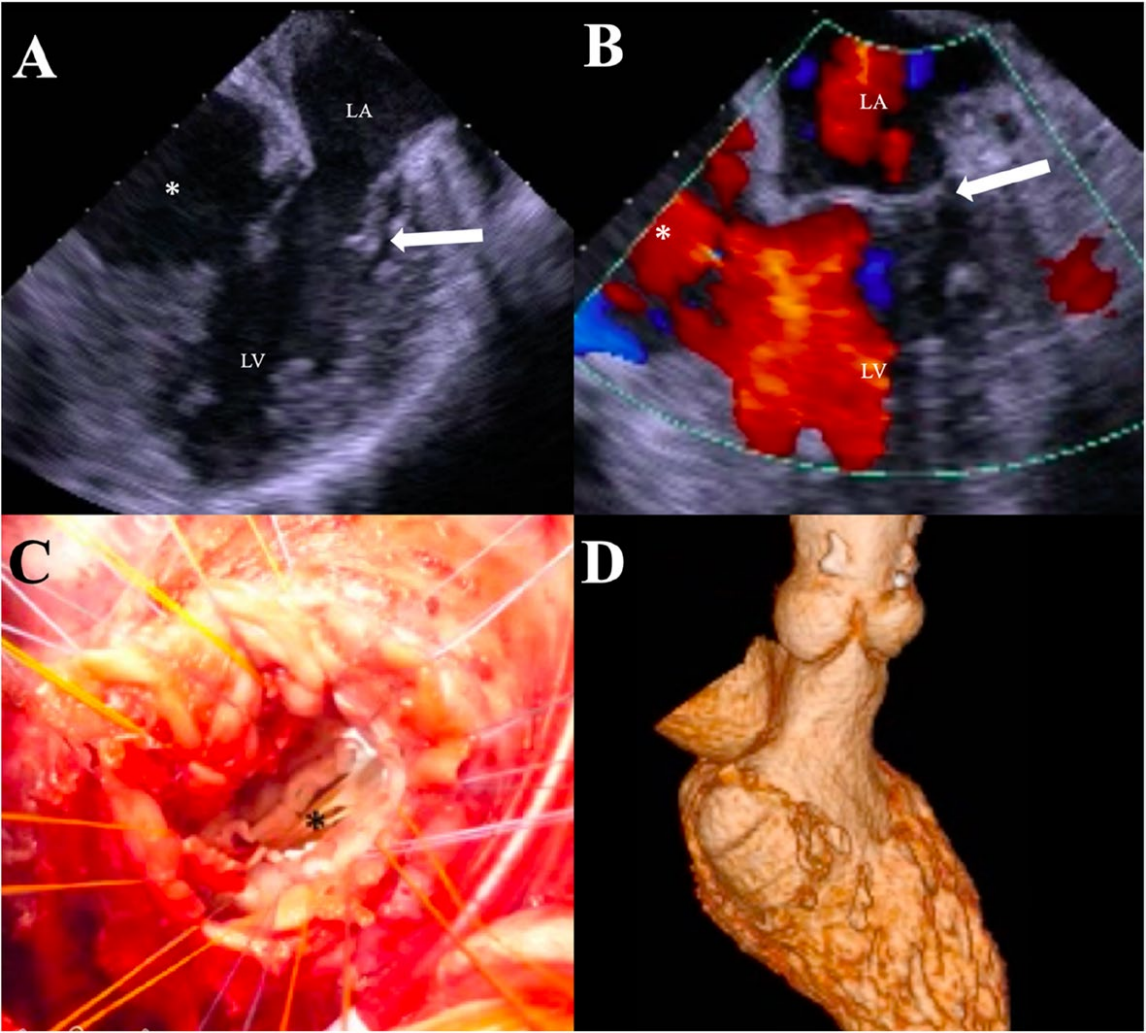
Transesophageal echocardiography revealed the left ventricular septal aneurysm (star) was near the mitral valve (arrow). A was diastole, and B was systole. C When the left ventricular septal aneurysm was incised, the entry port and left ventricular papillary muscle were confirmed (star). D Postoperative-enhanced CT revealed no pseudo-false aneurysm between left and right ventricle
